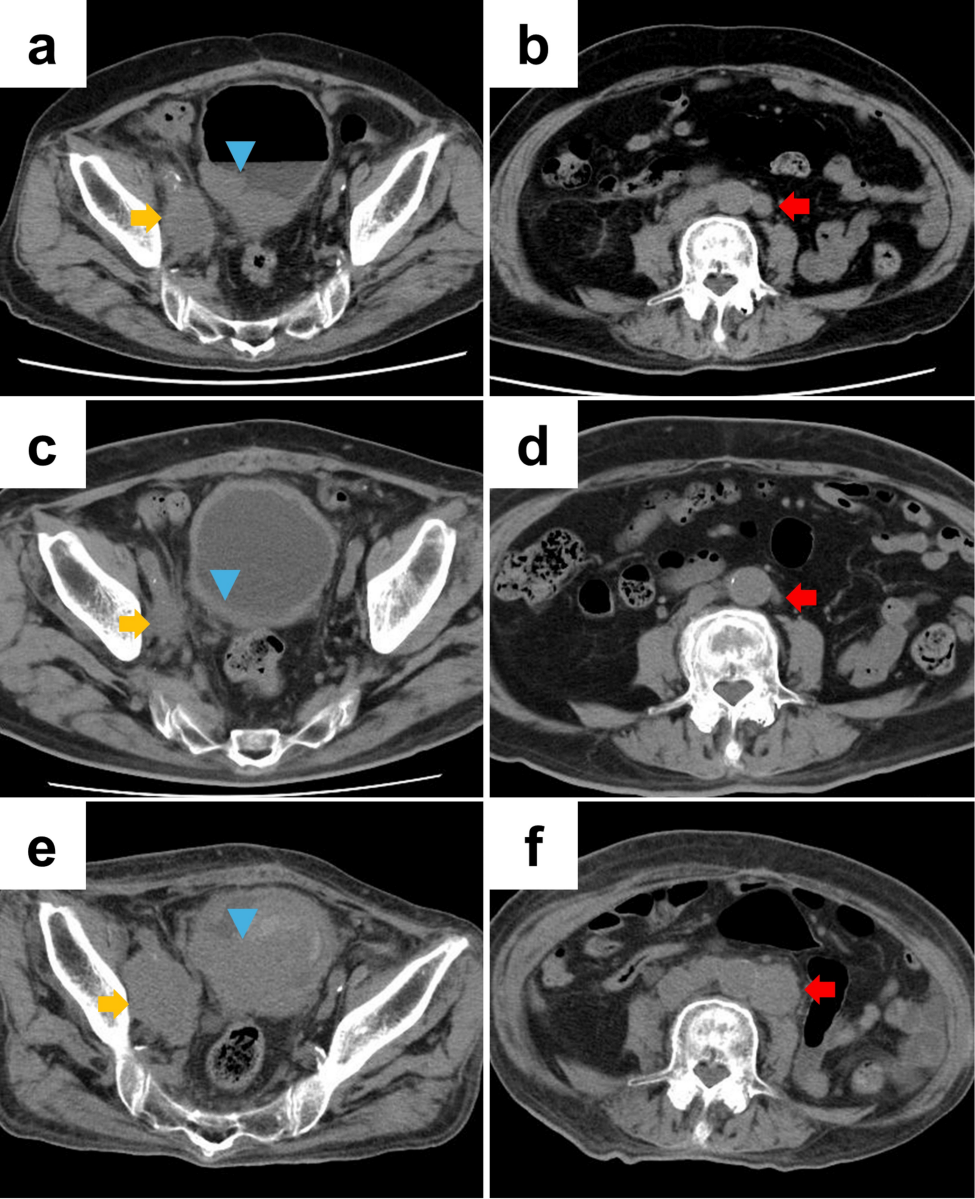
Computed tomography (CT) findings in Case 2 (a, b) Baseline contrast-enhanced CT at the time of diagnosis of metastatic disease. (a) Bladder tumor with suspected extravesical extension and right obturator lymph node metastasis. (b) Para-aortic lymph node metastasis. (c, d) CT after two cycles of first-line enfortumab vedotin plus pembrolizumab (EVP) showing reduction in the size of the bladder tumor, right obturator lymph node metastasis, and para-aortic lymph node metastasis. (e, f) CT at 5.2 months after initiation of EVP, demonstrating marked regrowth of the bladder tumor and both nodal metastases, consistent with radiologic disease progression. The blue arrowhead, yellow arrow, and red arrow indicate the bladder tumor, right obturator lymph node metastasis, and para-aortic lymph node metastasis, respectively.
